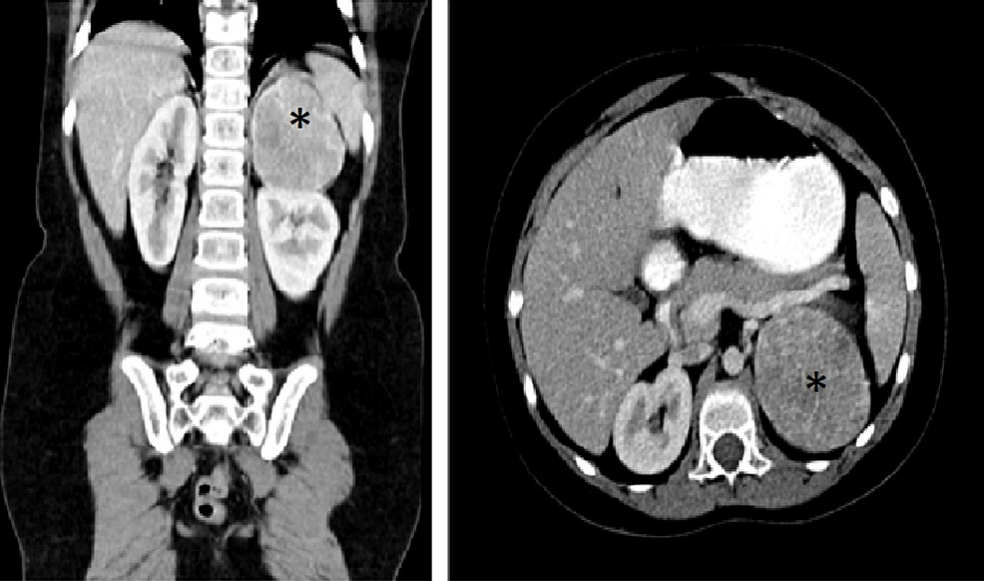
CT of the chest, abdomen, and pelvis detected a 5.5 × 6.4 × 5.3 cm left adrenal mass. CT: computed tomography
